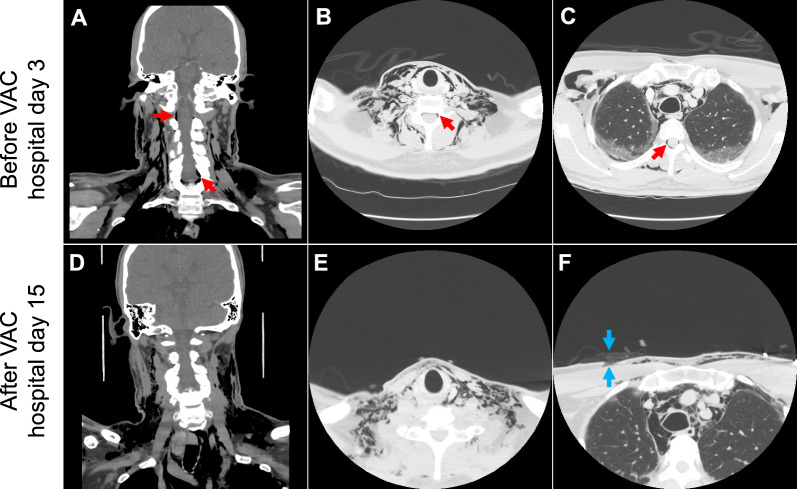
Computed tomography of the neck obtained before (A–C, on hospital day 3) and after vacuum-assisted closure therapy (D–F, on hospital day 15). (A–C) The images were obtained immediately after the patient developed a “popping sensation” in the neck, showing extensive subcutaneous emphysema tracking along the fat planes in the bilateral neck and chest. There was extensive pneumomediastinum and right apical pneumothorax. Air was also in the epidural space (red arrows). (D–F) After VAC therapy, the overall volume of superficial emphysema throughout the neck decreased. The right anterior chest blowhole incision is seen connected to topical vacuum-assisted closure dressing (blue arrows). (A and D), coronal section; (B, C, E, and F), axial section
